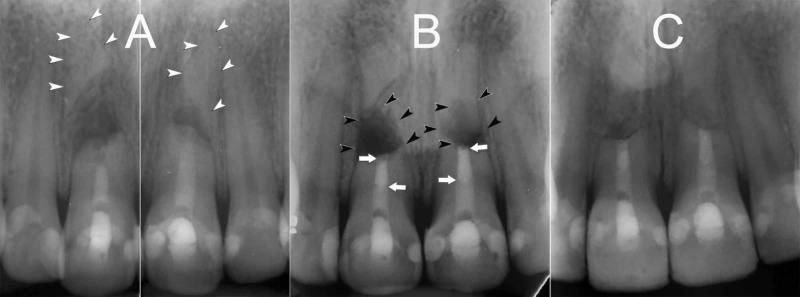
(A) Preoperative periapical radiographs of the fractured maxillary central incisors. (B) Without removing the apical fragments, 3-mm deep root-end cavities on the coronal segments were filled with CEM cement. (C) 30-month recall radiograph; satisfactory healing of the lesions and normal PDLs can be seen (White arrow head = apical segment, Black arrow head = lesion, White arrow = root end filling with CEM). CEM: Calcium-enriched mixture
